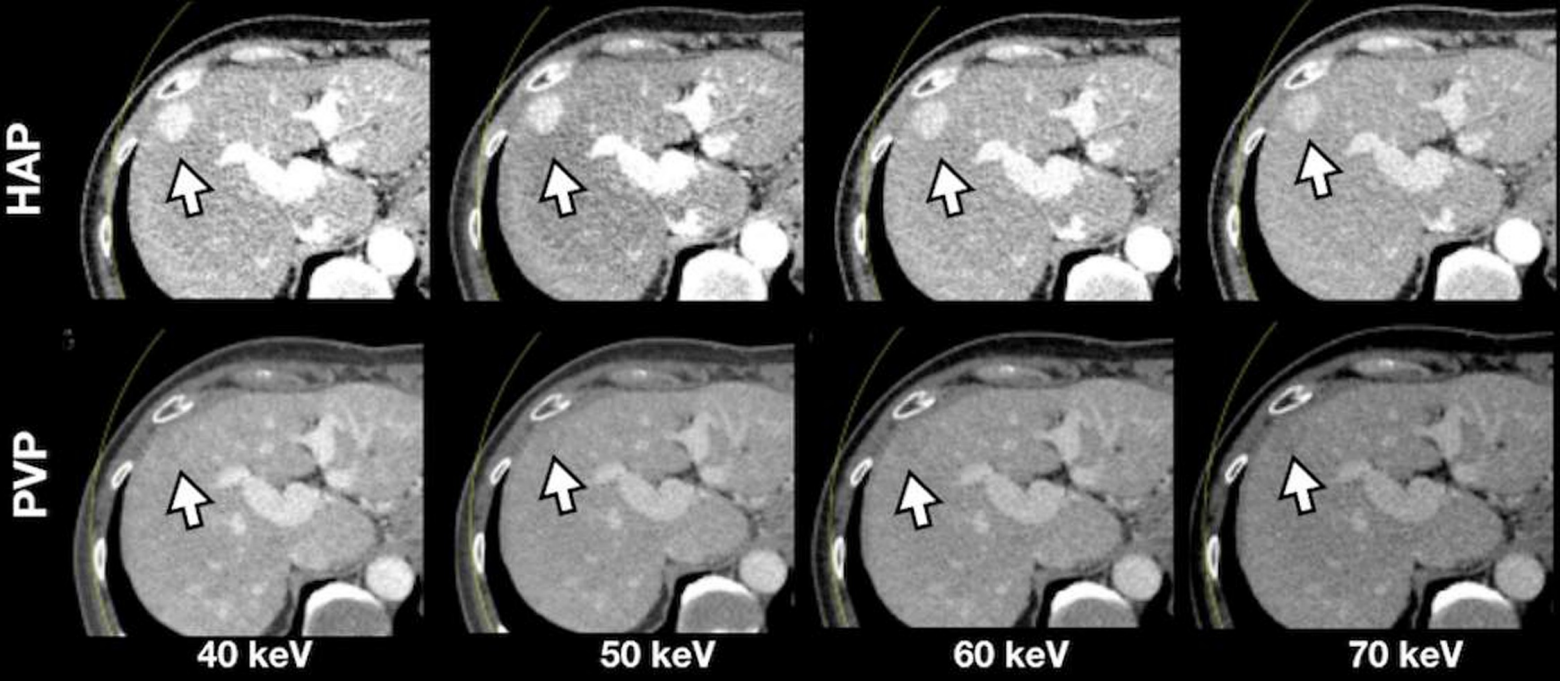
A representative case of a 68-year-old man with local tumor progression. A 68-year-old man (body weight, 70.4 kg; BMI, 24.1 kg/m^2^) underwent multiphasic hepatic CT with dual source DECT at half reduced contrast medium dose. The image was reconstructed with nMERA images at 40–70-keV. All the images were under optimized window width/center setting to each image. The conspicuity of the hypervascular HCC (arrows) gradually improves as the energy level decreases in nMERA images, especially in the HAP image.
